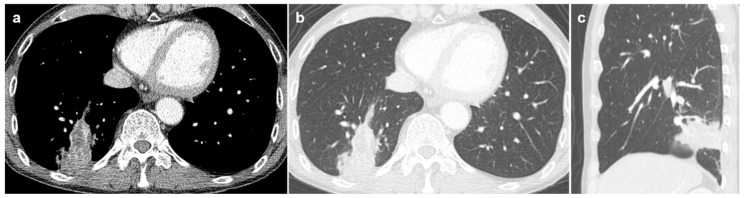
A male patient with nontuberculous mycobacterial infection. (a–c) Chest CT shows a focal patchy consolidation with internal low attenuation in right lower lobe, which had persisted for two months. Percutaneous core needle biopsy of the lesion revealed chronic granulomatous inflammation with necrosis. Subsequent bronchial lavage fluid culture was positive for NTM.
